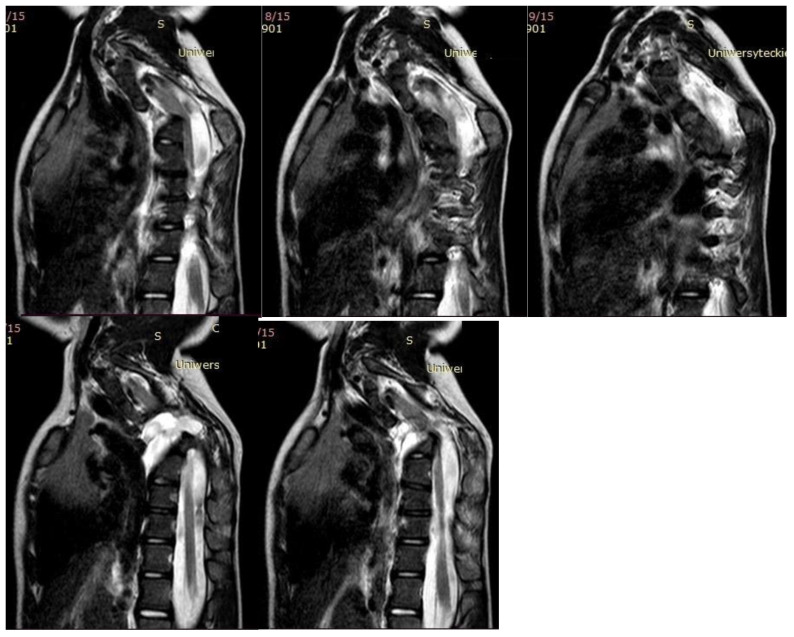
MRI of the five-year-old girl before surgical treatment.
